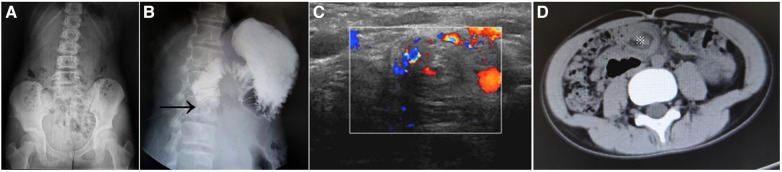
Plain abdominal film showed scant intestinal gas (A). The UGIs demonstrate obstruction of the second part of the duodenum (arrow) (B). Ultrasound shows the “whirlpool” sign, suggestive of malrotation with volvulus of 270°−360° (C). Axial CT image shows the “whirlpool” sign (asterisk) (D). UGIs, upper gastrointestinal series; CT, computed tomography.
